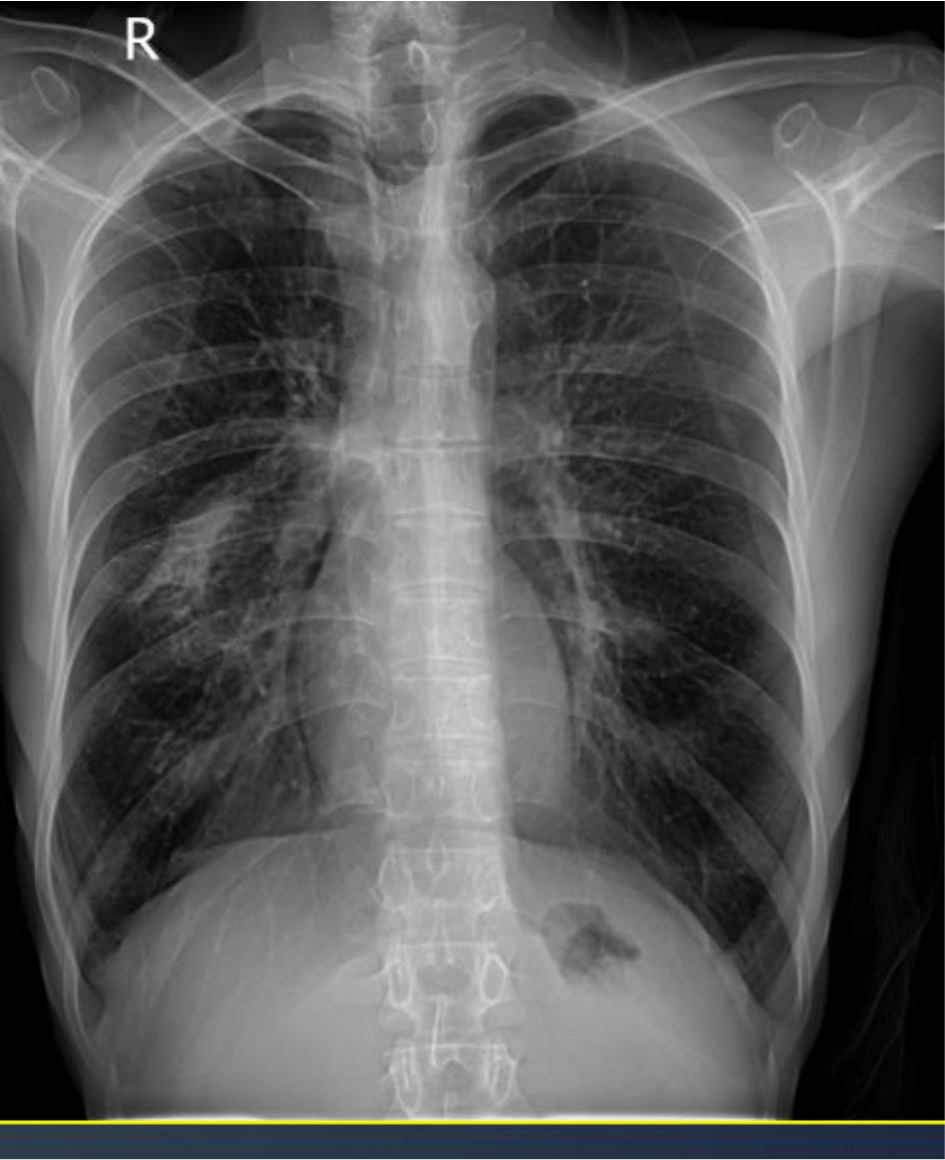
Follow-up chest X-ray showed significant improvement after 2 months of treatment, but the infiltration hadn’t been completely resolved
